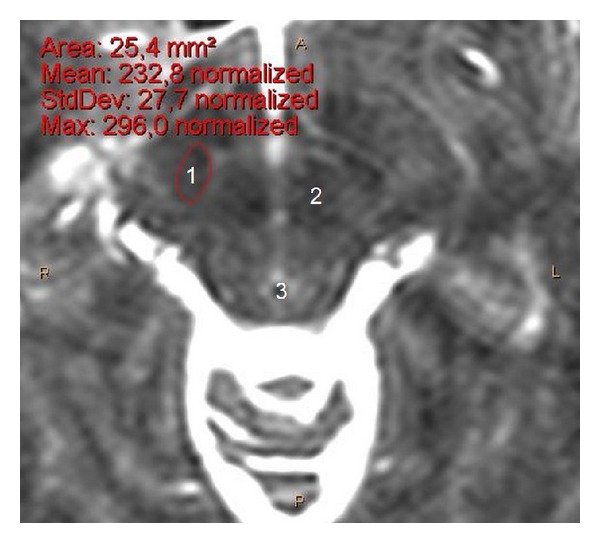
Transverse cerebral MRI section (T2-weighted, 1.5 Tesla) at level *Z* = −4 (zoom on mesencephalon), from a 37-year-old male, showing the STN location and surface area. 1: STN, 2: red nucleus, 3: aqueduct (of Sylvius), A: anterior, P: posterior and, R: right, L left.
